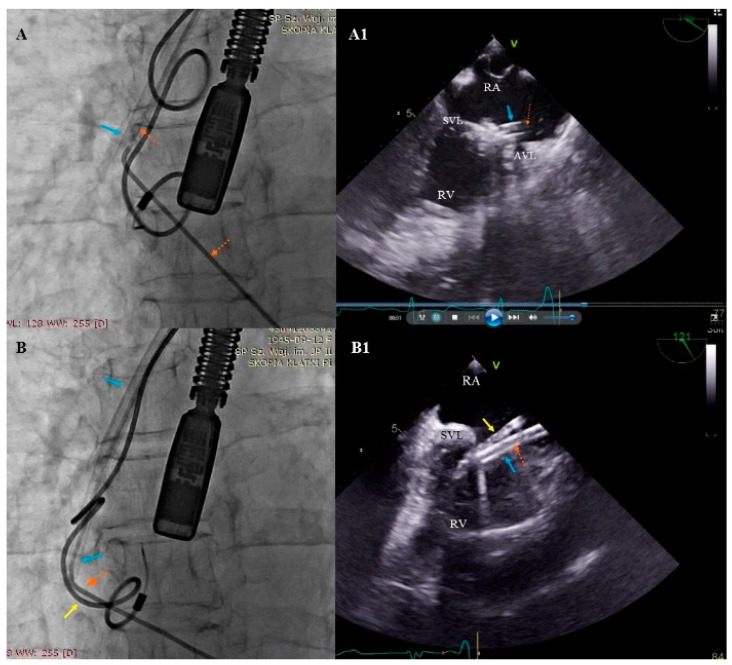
Winding of the leaflet on the dilatator while removing the ventricular lead (simultaneous images from fluoroscopy and transesophageal echocardiography (TEE). (A,A1) Tight ventricular lead (red arrow) and Byrd’s dilatator (blue arrow) winding the valve leaflets. (B,B1) When rotating the dilator in one direction (blue arrow), winding of the tricuspid valve (TV) leaflet and the attached atrial lead (yellow arrow) occurred (as a result of adhesions of the lead with the leaflet and inter-lead adhesions).
